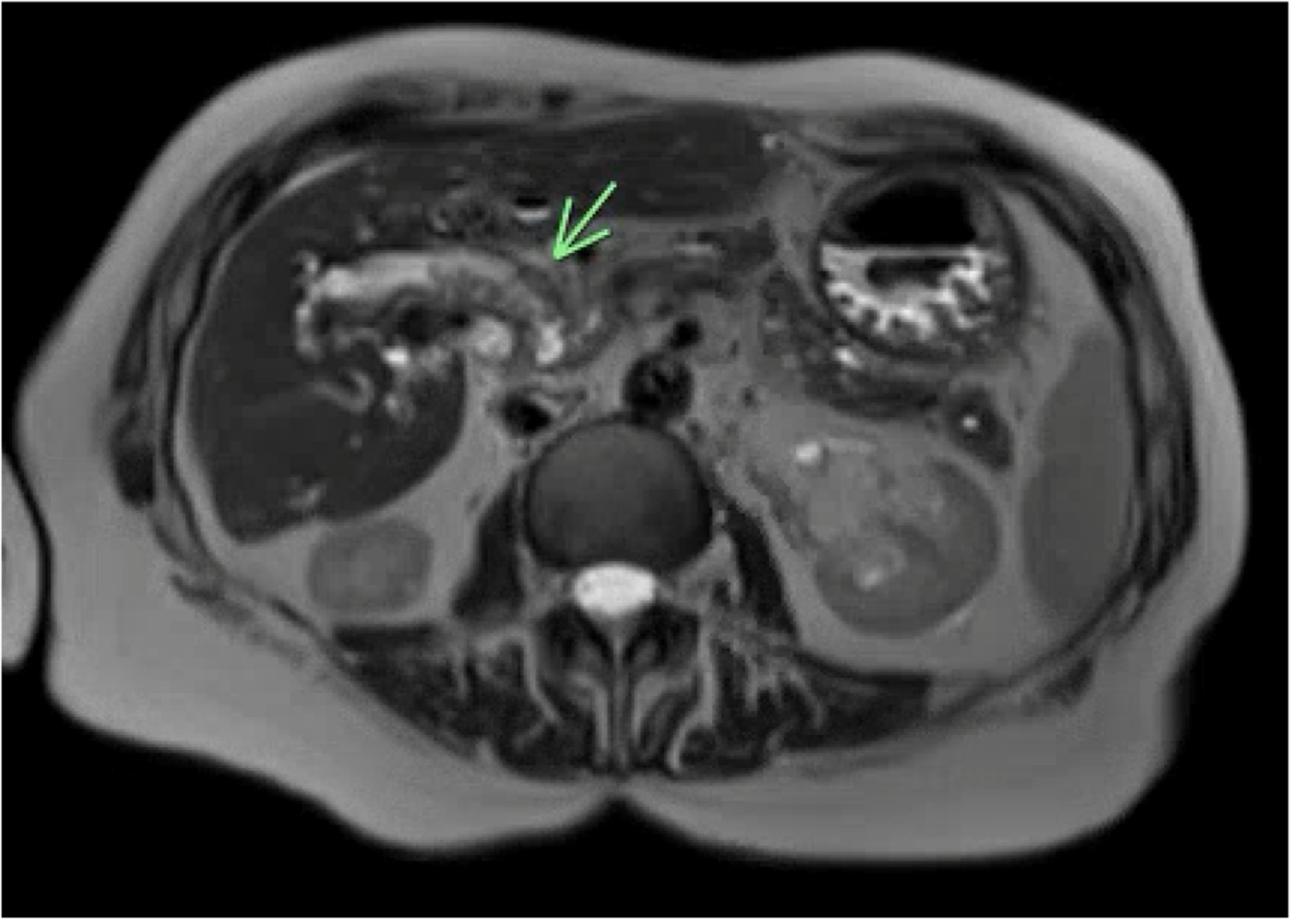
Posttreatment magnetic resonance imaging 6.5 months after treatment (axial T2 sequence) demonstrating interval resolution of the hepatic mass, but findings were concerning for ascending cholangitis.
